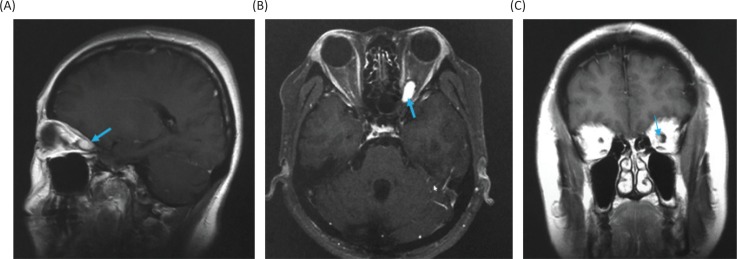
Gadolinium-enhanced magnetic resonance images at 18 months after the stereotactic radiosurgery. (A) Sagittal image. (B) Axial image. (C) Coronal image.
